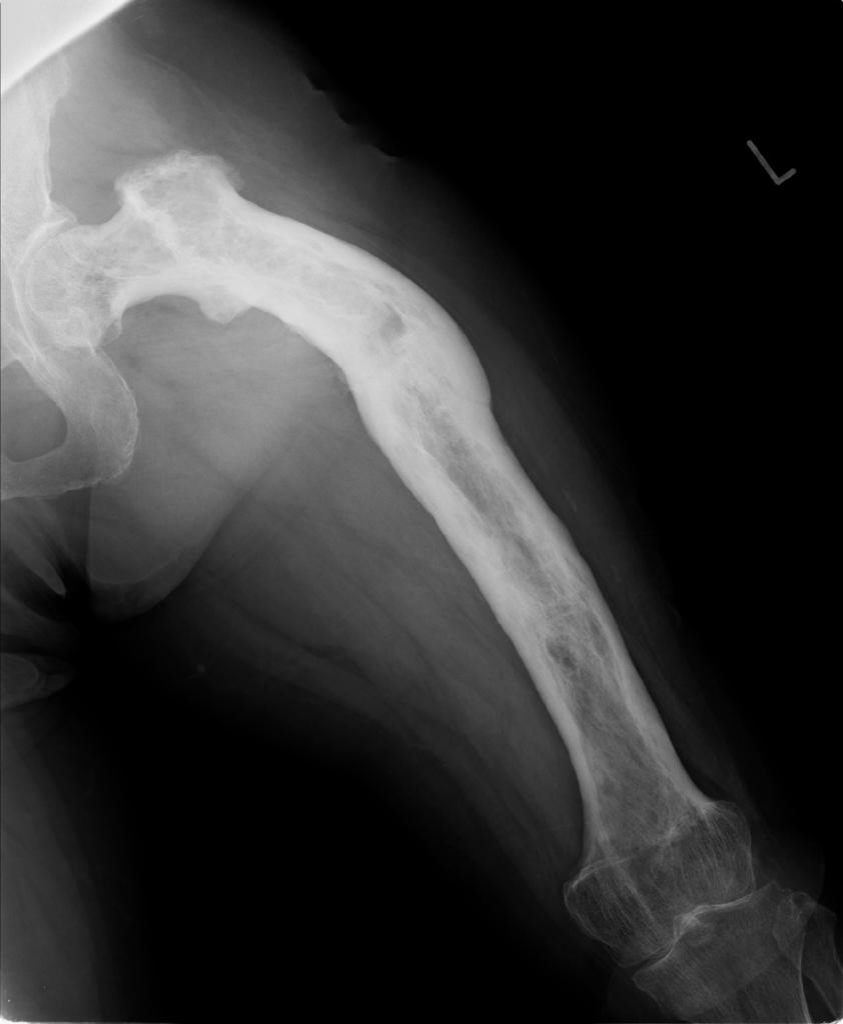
Anteroposterior radiographic view 5 year after injury.
